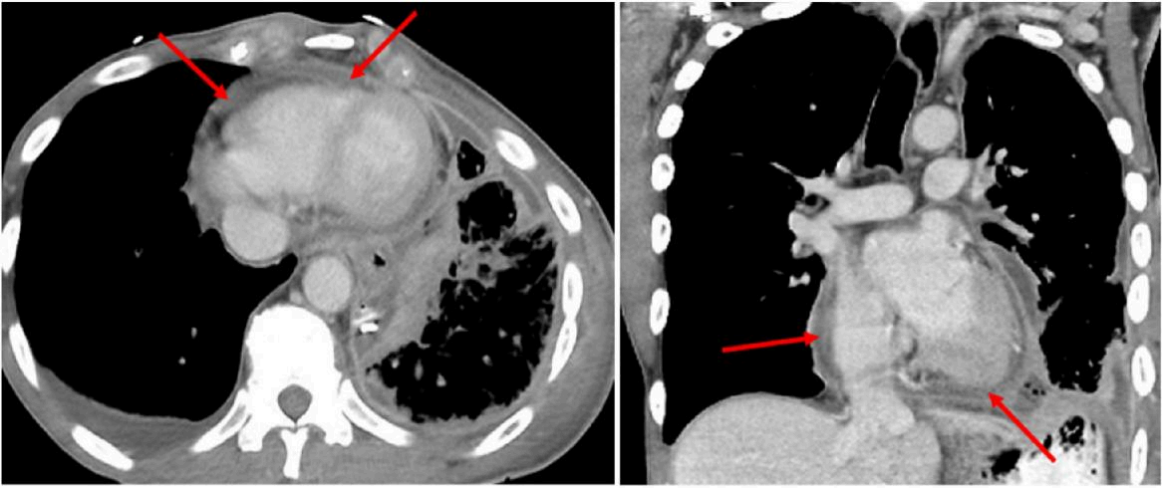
Chest computed tomography after repair of oesophageal perforation (axial and coronal computed tomography). Thickened and inflamed pericardium and pericardial fat (red arrows).
